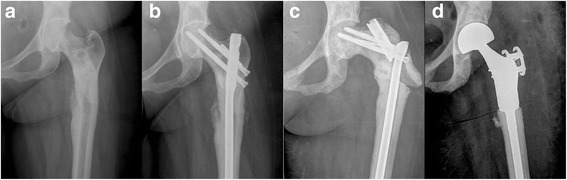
The clinical course of one patient who undergone revision surgery after implant failure. X-ray imaging revealed an impending fracture and an osteoblastic lesion in the left subtrochanteric part of femur (a). IMN was performed, and the postoperative course was uneventful until the implant failure at the 50-month clinical follow-up (b). X-ray imaging showed a broken implant in proximal part (c). The IM rod was removed and the proximal part of the femur was resected and then replaced with EPR (d)
